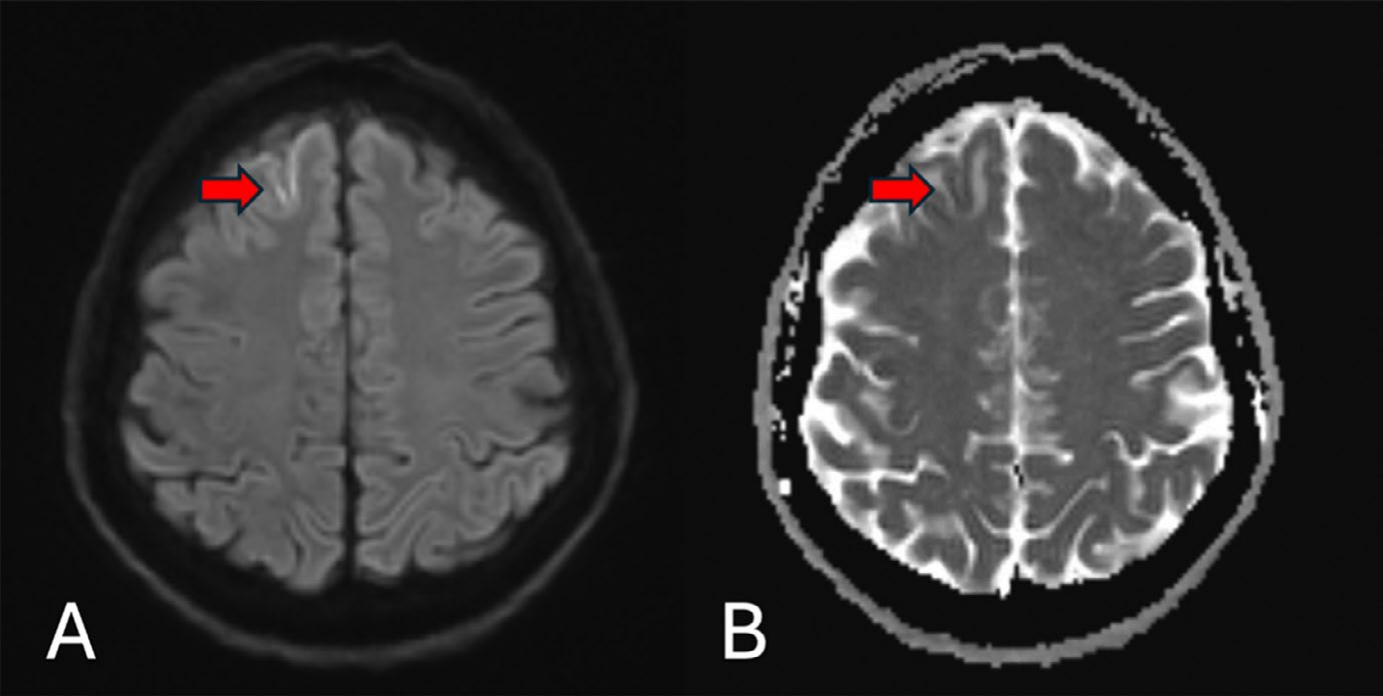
(A) DWI axial MRI and (B) ADC axial MRI showed gyriform pattern diffusion restriction (the red arrows).
